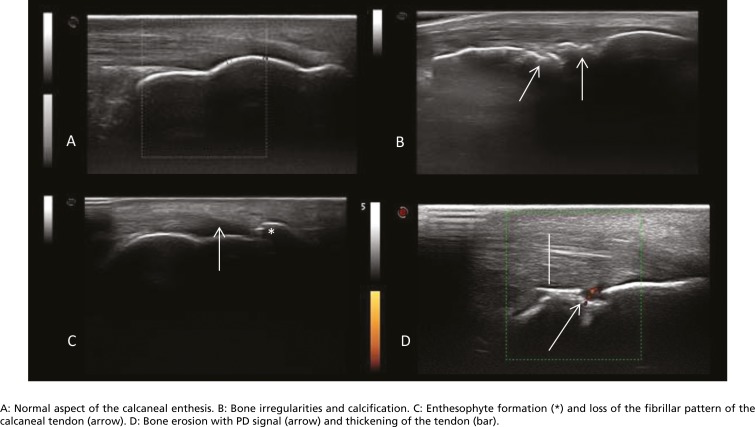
Ultrasound images of the calcaneal enthesis.
